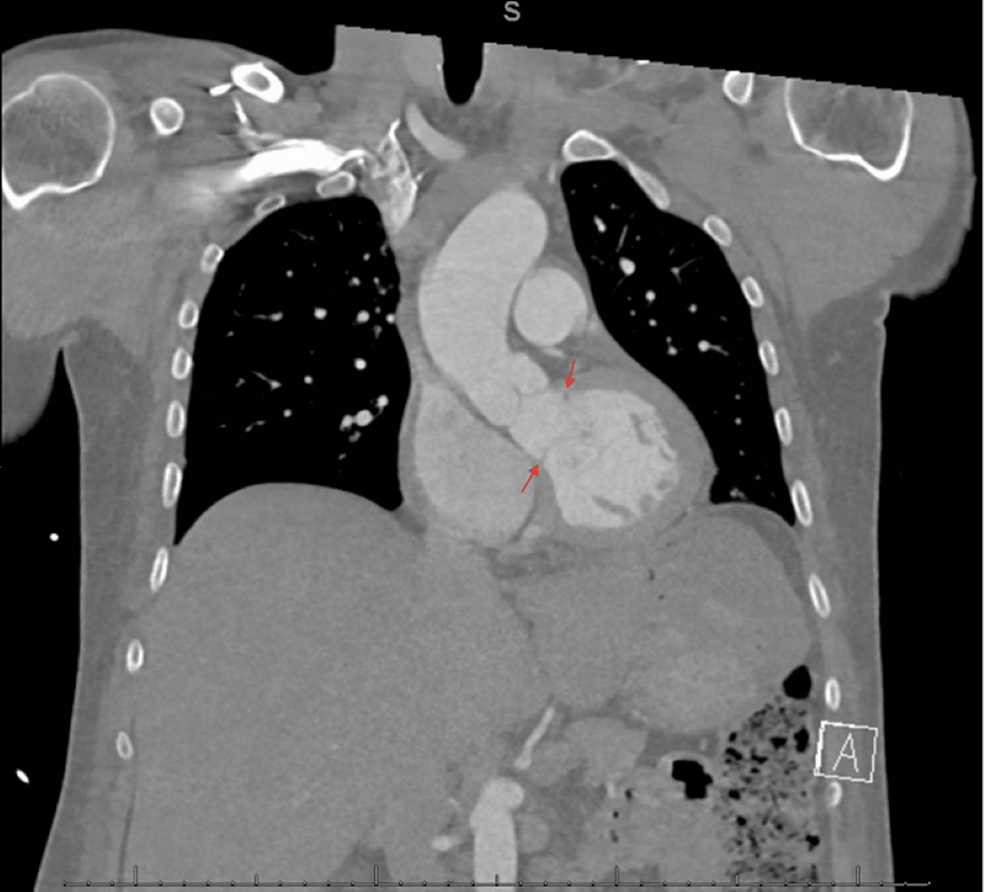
Patient’s CT Chest demonstrating LVOT obstruction. LVOT: left ventricular outflow tract
